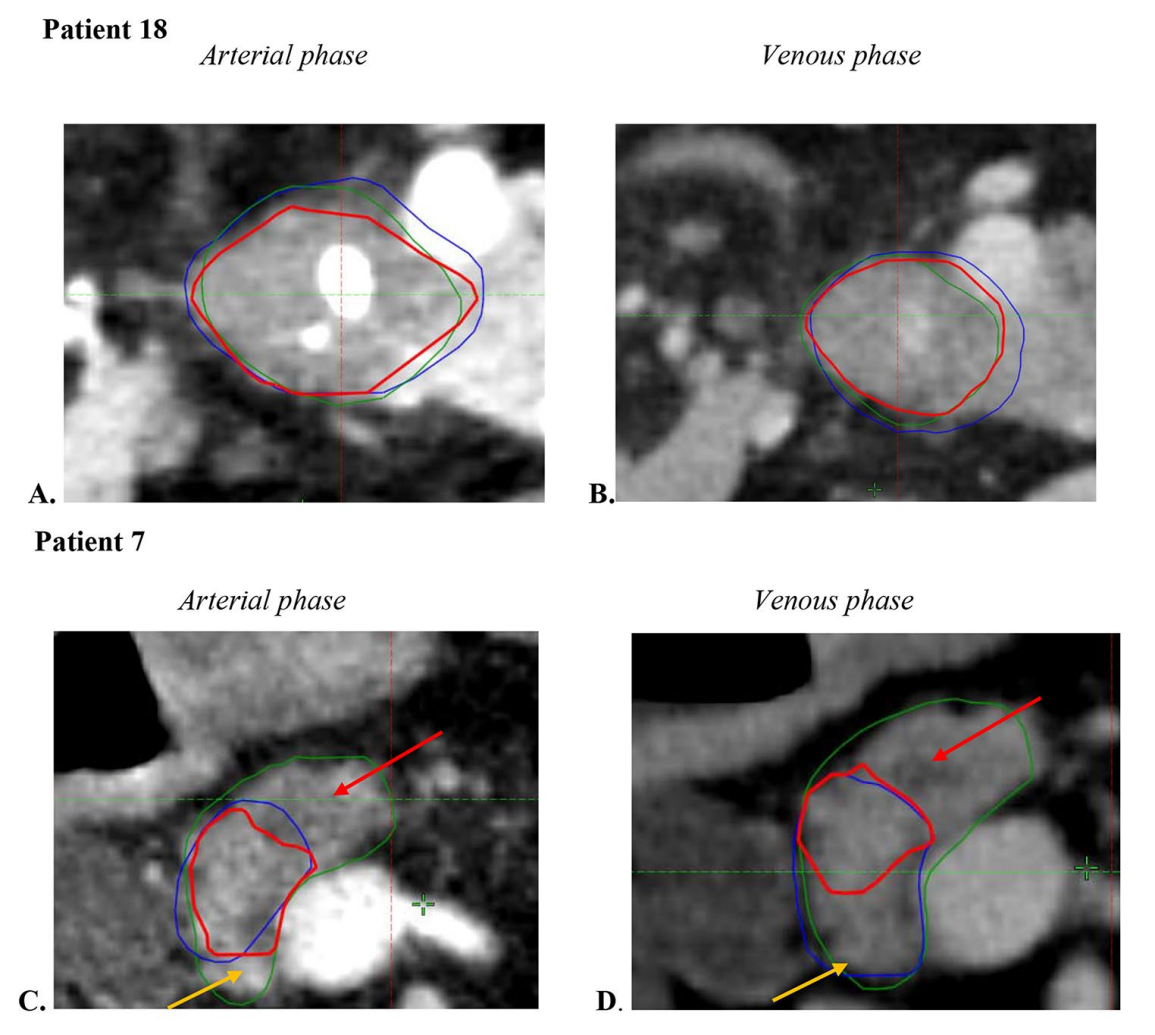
Representative axial CT scans showing inter-observer variability during delineation of the GTV in two patients. Patient 18 (A, B) represents the best JCI case (hypodense tumor with good concordance). Patient 7 (C, D) represents the worst JCI case (red arrow: iso-attenuating tumor with dilated bile duct; yellow arrow: normal pancreactic parenchyma) gsGTVs are shown in red; radiation oncologists’ GTVs are shown in blue and green at the arterial (A, C) and the venous phases (B, D)
